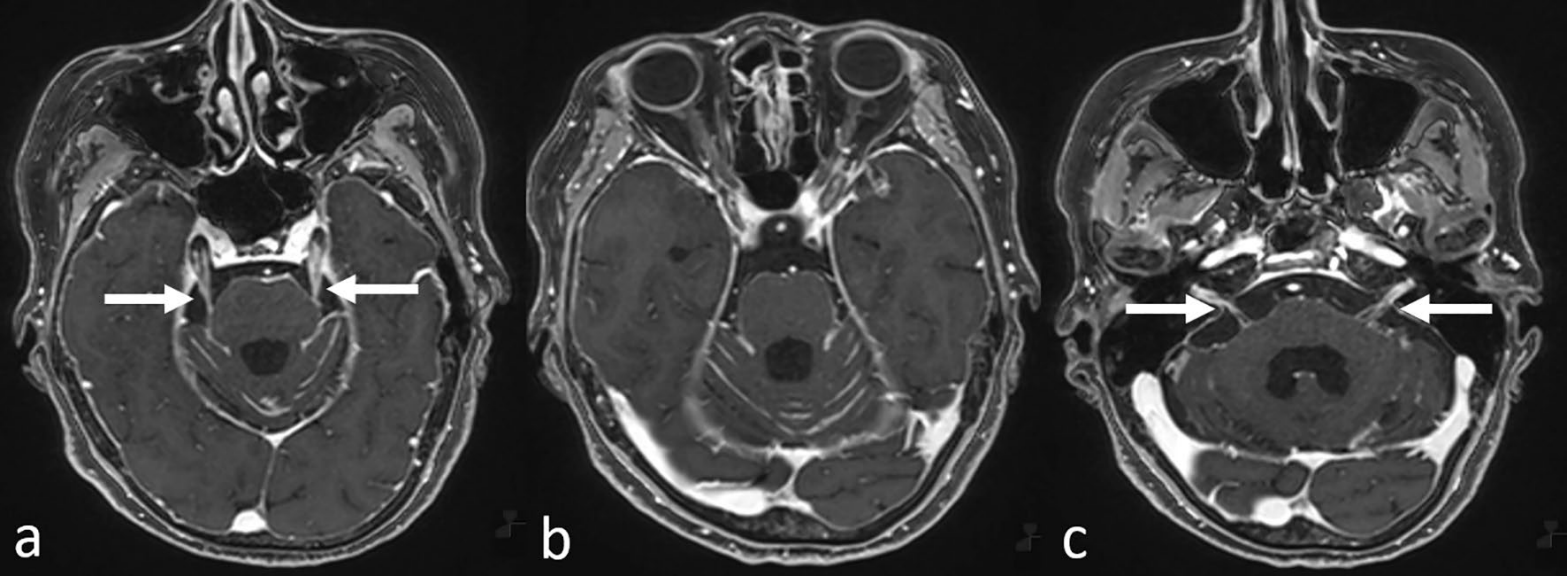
Leptomeningeal carcinomatosis in a patient with gastric cancer. Contrast-enhanced 3D fat-suppressed T1-weighted images show enhancement in the bilateral cranial nerve V (a, arrows), brain stem and cerebellar meninges (a–c), and bilateral internal auditory canal corresponding to cranial nerves VII/VIII (c, arrows)
